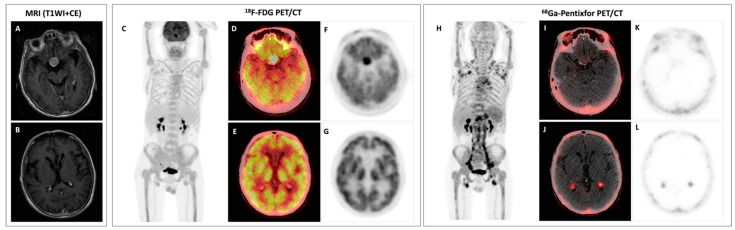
Baseline evaluation, axial contrast-enhanced T1-weighted MRI (A,B), MIP image of the ^18^F-FDG PET (C), axial fusion ^18^F-FDG PET/CT (D,E), axial ^18^F-FDG PET (F,G), MIP image of the ^68^Ga-Pentixafor PET (H), axial fusion ^68^Ga-Pentixafor PET/CT (I,J), and axial ^68^Ga-Pentixafor PET (K,L). This patient (male, 63 years old) had a numbness and amyosthenia in the right foot 2 months prior, and his symptom progressively developed to the bilateral lower and upper extremities. Electromyography showed demyelination and axonal injury of sensorimotor peripheral nerves in the lower extremities. Laboratory tests showed anemia (hemoglobin 94 g/L) and elevated serum IgM (30.49 g/L; reference: 0.4–2.3 g/L). Serum protein and immunofixation electrophoresis were positive for monoclonal protein (18.5 g/L, IgM-κ). Bone marrow biopsy showed infiltration with lymphoplasmacytoid cells accounting for 10% of the cells in bone marrow. Therefore, the diagnosis of Waldenström’s macroglobulinemia (WM), a low-grade lymphoma, was established. Since the patient had a chief complaint of peripheral neuropathies, Bing–Neel syndrome (central nervous system involvement in WM) was considered, as other common causes of peripheral neuropathies in WM—paraprotein-related peripheral neuropathies, cryoglobulinemia, light chain amyloidosis—were excluded by sural nerve biopsy and negative serum cryoglobulin. The cerebrospinal fluid examinations revealed an M-spike of 0.19 g/L (IgM-κ restricted) and positive mutation of MYD88^L265P^ (an important marker for diagnosing WM [1,2]), supporting the diagnosis of Bing–Neel syndrome. The patient then underwent a brain MRI, which was unremarkable except for a pituitary macroadenoma (A). In ^18^F-FDG PET/CT, the pituitary macroadenoma was hypermetabolic ((D,F), SUVmax 10.4); additionally, diffusely and homogenously increased uptake in bone marrow and involvement in lymph nodes were also depicted (C). The patient then underwent ^68^Ga-Pentixafor PET/CT, a chemokine receptor CXCR4-targeted imaging, which we previously reported as being superior to ^18^F-FDG in patients with WM [3]. MIP image of the ^68^Ga-Pentixafor PET showed markedly increased radioactivity throughout the axial and appendicular skeleton, as well as multiple lymph nodes in the neck, axilla, para-aortic, pelvic, and inguinal areas with increased uptake (H).Surprisingly, ^68^Ga-Pentixafor PET/CT showed active tracer uptake in bilateral choroid plexus ((J,L), SUVmax 4.0) that was negative with ^18^F-FDG (E,G) and also with normal signal intensity and contrast-enhancement in MRI (B); the FDG-avid pituitary macroadenoma was negative with ^68^Ga-Pentixafor (I,K). Furthermore, involvements in the thoracic nerve root and sacral nerve root were also detected.
